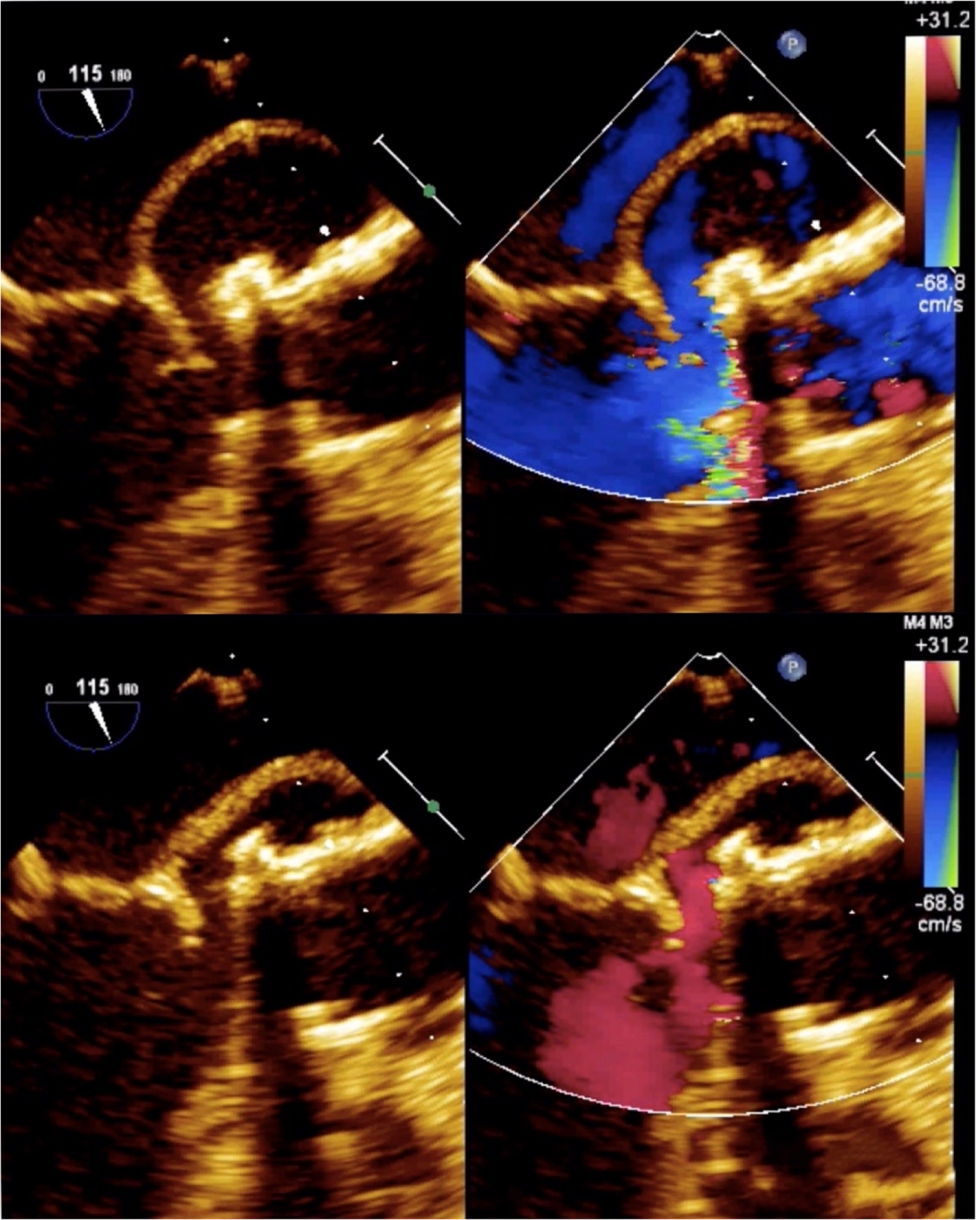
Transesophageal echocardiogram confirming the presence of the subannular anechoic formation with the appearance of internal flow signal at the color Doppler evaluation, suggesting the communication with the remaining periprosthetic sac, compatible with a refurbished pseudoaneurysm
